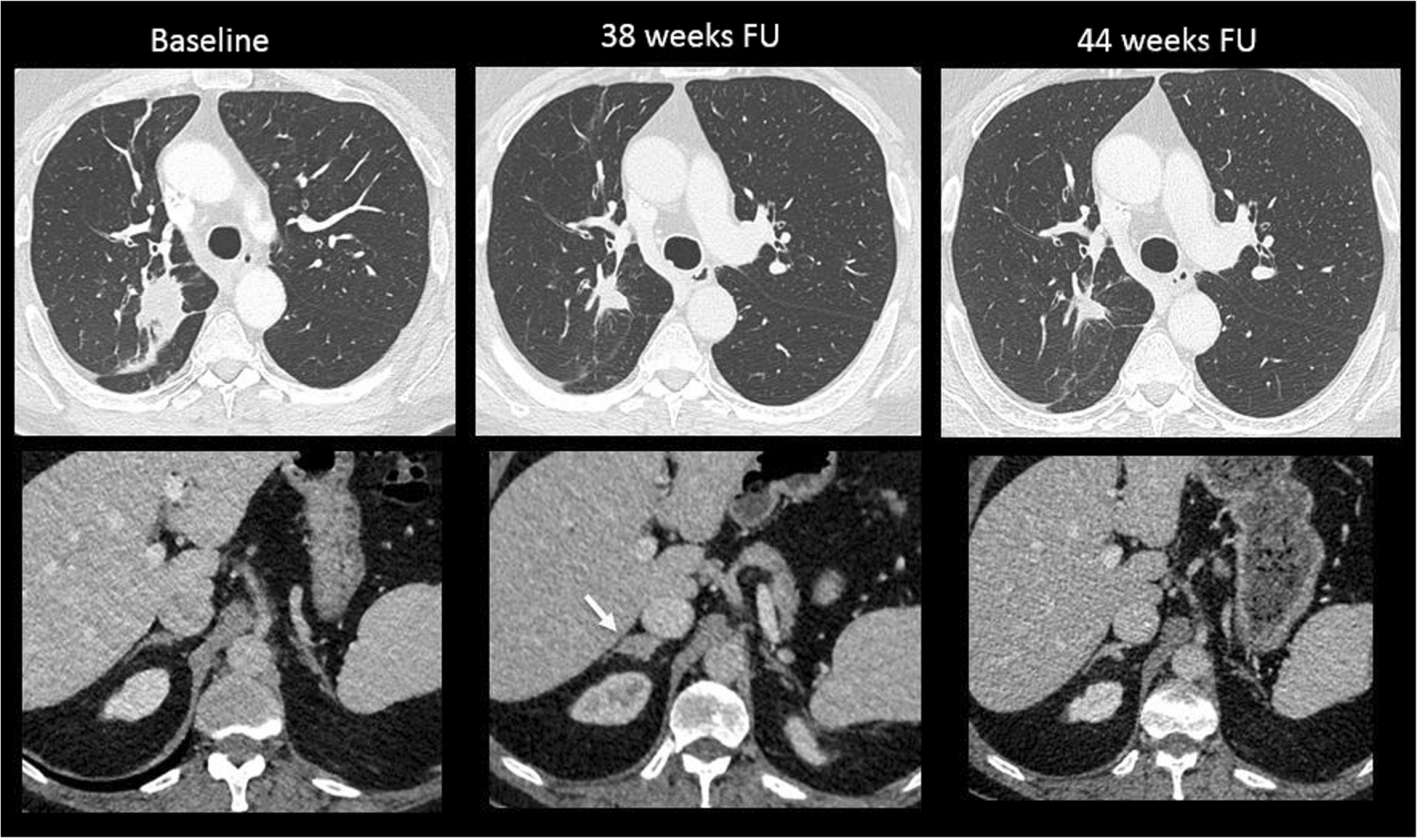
Pseudoprogression in a 65-year-old patient with lung carcinoma treated with nivolumab (anti-PD-1). Baseline axial CT showed a lung mass in the upper right lobe with normal adrenal glands. At a 38-week follow-up (FU), there was a good reduction in the size of the lung mass, but a new lesion appeared in the right adrenal gland (arrow). The patient was maintained under the same treatment. At 44-week follow-up, the right adrenal mass disappeared, confirming the diagnosis of pseudoprogression
